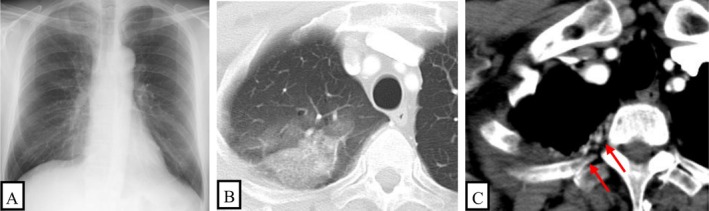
(A) Chest radiography showed decreased lucency in the right lung apex. (B) Axial images from CTA showed ground‐glass opacities and consolidation in the right upper lobe, consistent with aspirated blood. (C) Focal pleural thickening (3 mm) with enhancing vascular structures (red arrow) was observed in the right lung apex.
